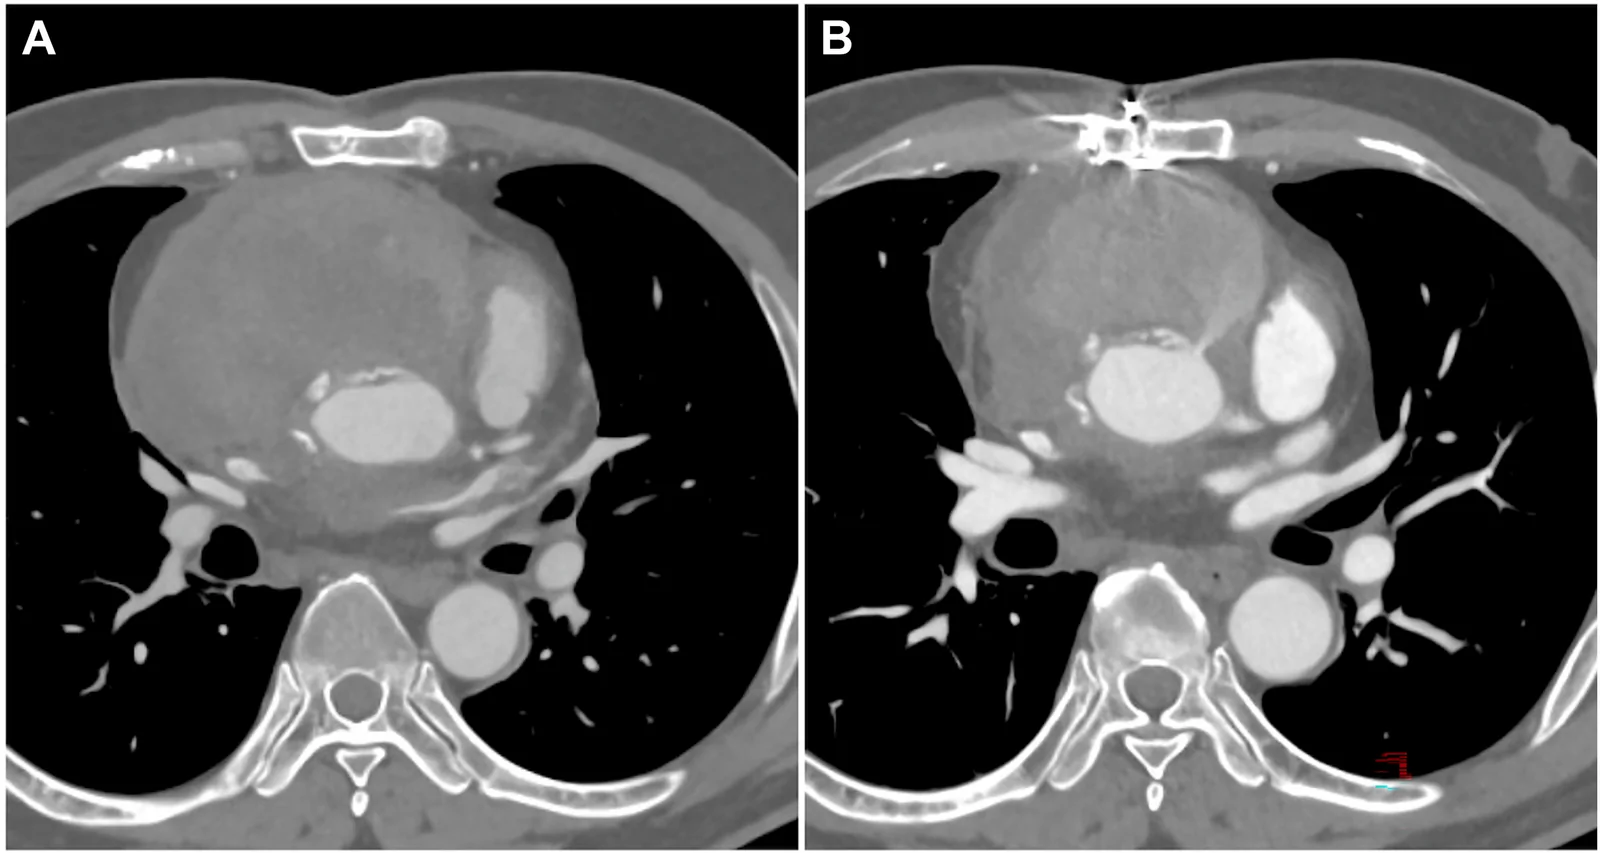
(A) Admission contrast-enhanced computed tomography demonstrates a mediastinal hematoma and large ascending aortic pseudoaneurysm (107 × 59 mm). (B) Retrospective review of a computed tomography scan obtained 4 months earlier depicts a preexisting pseudoaneurysm with evident extravasation of contrast material originating from the proximal anastomotic site.
